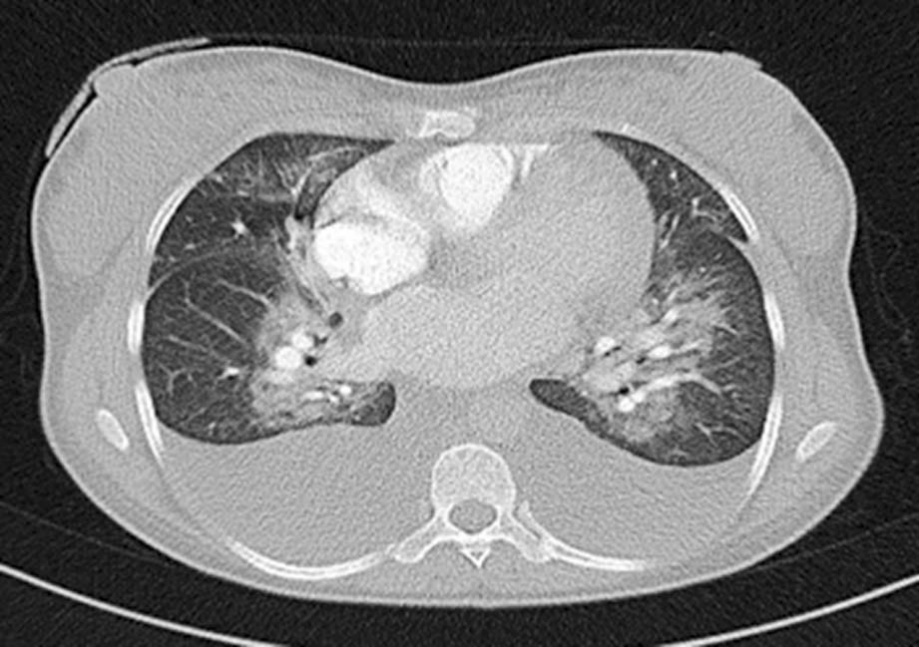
Computed tomography scan of the chest demonstrates bilateral pleural effusion.
